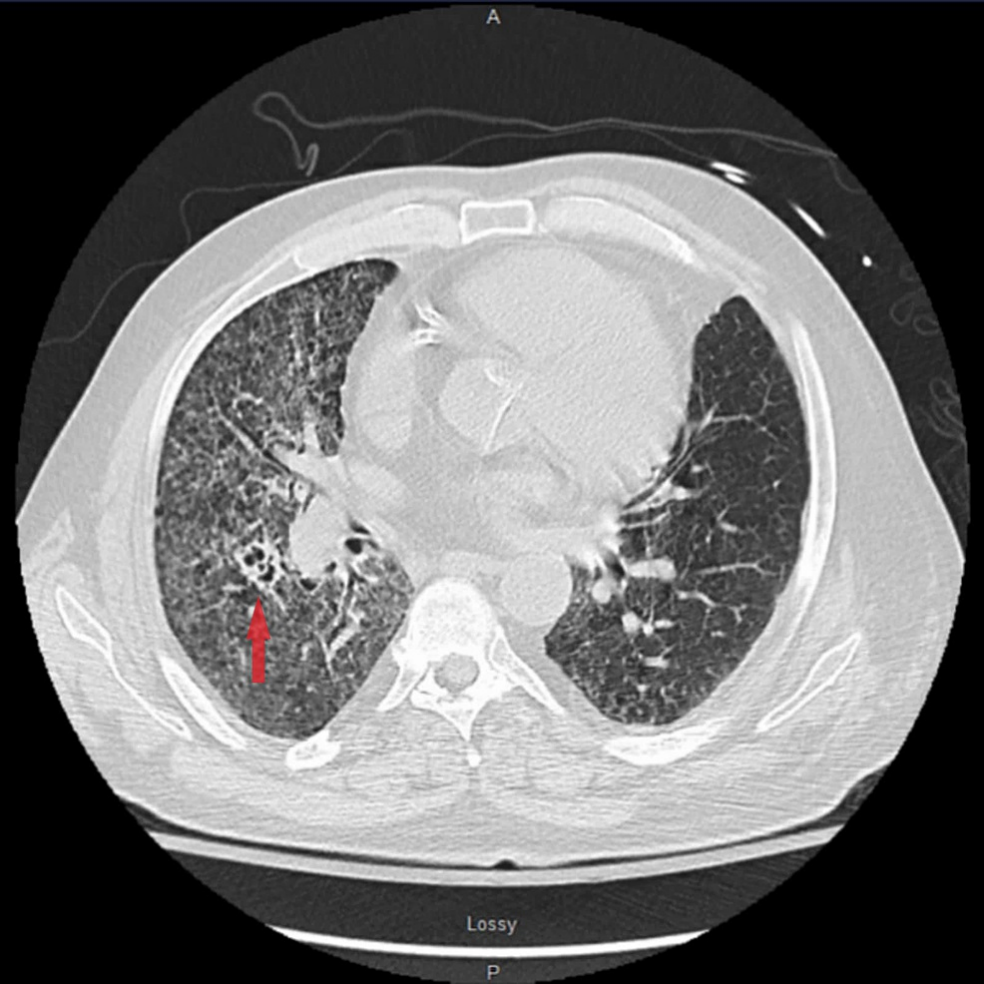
CT lungs showing diffuse micronodular changes in right lung in disseminated histoplasmosis (arrow) CT: computed tomography
